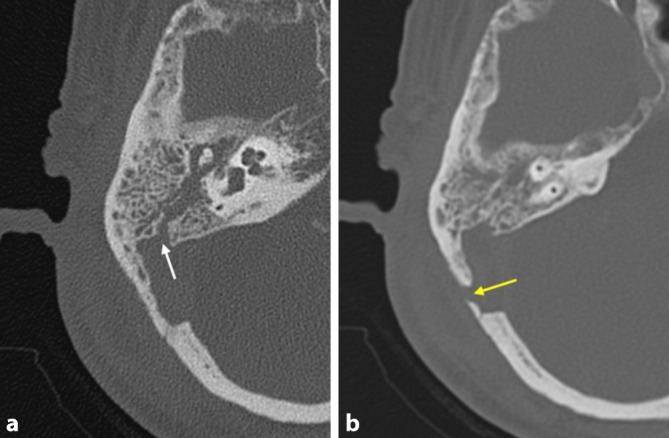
Axiale Computertomographie (CT). Rechtsseitige abszedierende Mastoiditis mit a dorsalem Kortikalisdefekt der Mastoidzellen (*weißer Pfeil*) und b knöchernem Defekt dorsal der Mastoidzellen (*gelber Pfeil*) mit Fortleitung der Entzündung nach extrakraniell
